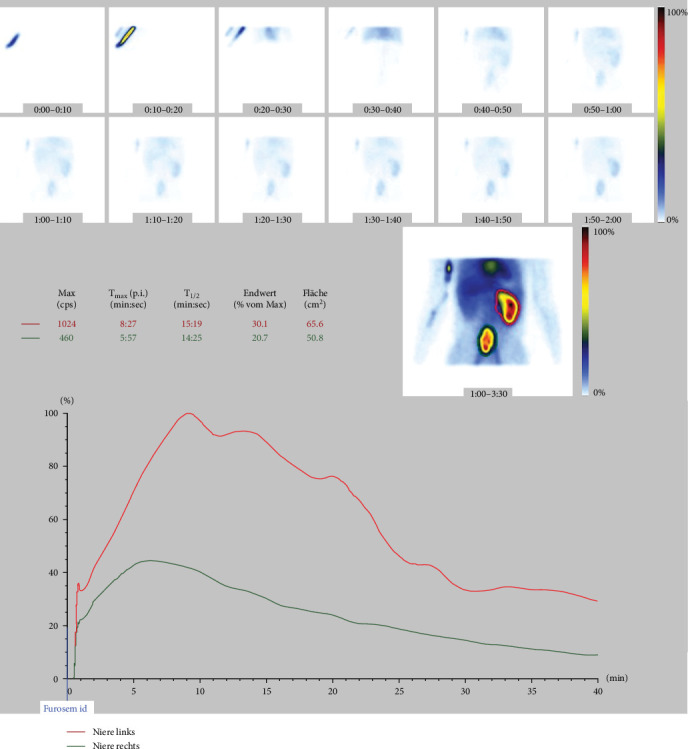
MAG3 scintigraphy showing prolonged clearance of the radiotracer, which, along with the clinical presentation, sonographic findings, and MRI scans, further supported the clinically suspected diagnosis of UPJO of the right kidney.
